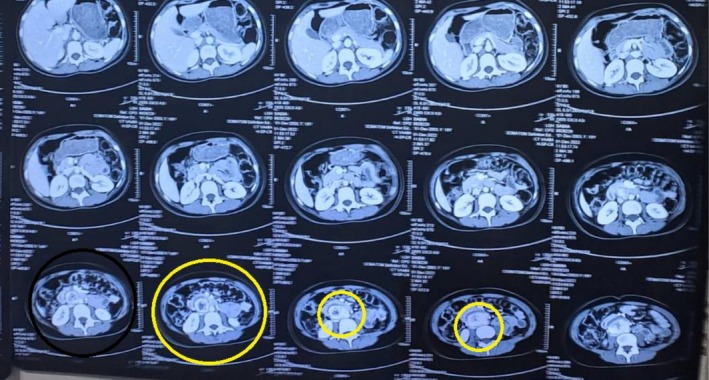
The encircled section in CT Abdomen with IV contrast showing the mass.
